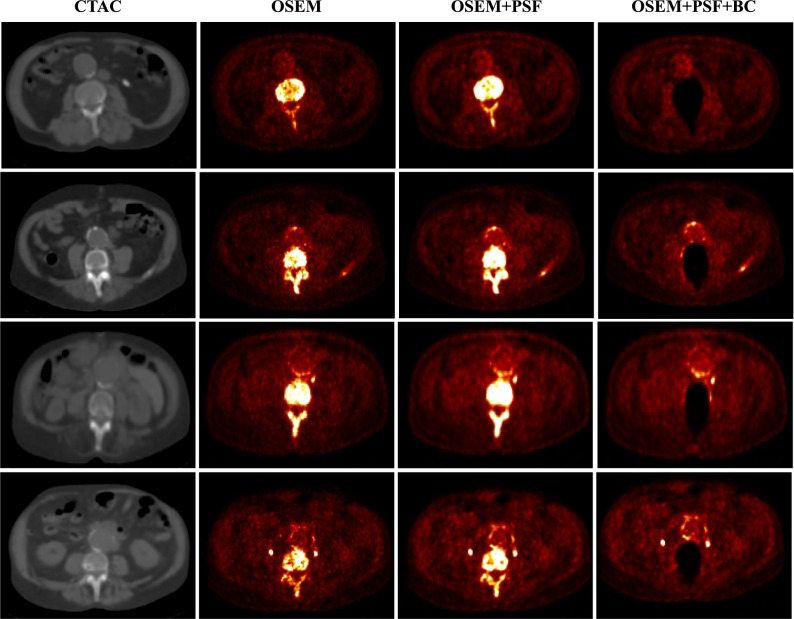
CT images and PET reconstructed images of four indicative patient datasets, showing a high [^18^F]-NaF uptake in the bone and the aneurysm. The activity contribution from the bone was removed in OSEM + PSF + BC
